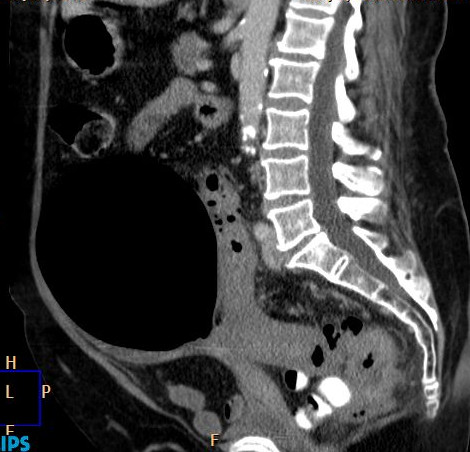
A giant colonic diverticulum on computed tomography.
